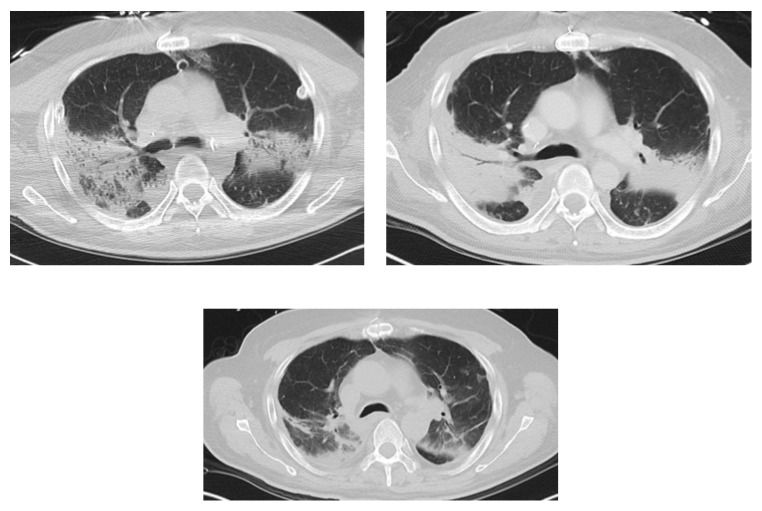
Serial chest CT imaging prior to and after treatment with amphotericin. The first image shows the patient's chest at day +12 after transplantation with significant disease in the dependent areas of the lungs bilaterally that has progressed to dense consolidation by day +25 (3 days prior to diagnosis) as the second figure shows while the third image shows the patient's chest imaging at day +90 with significant improvement.
